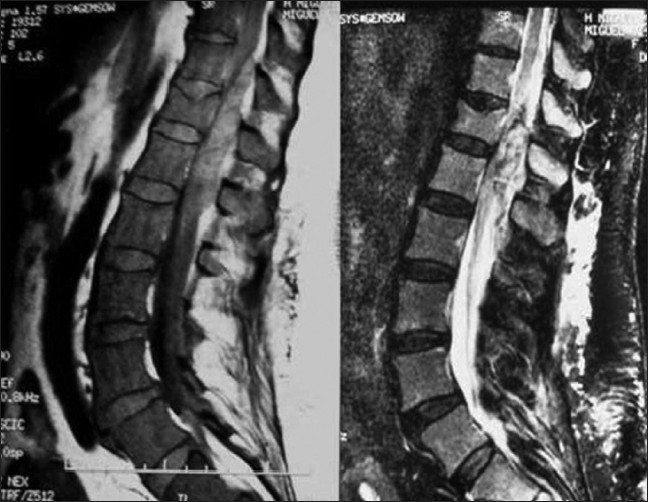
Sagittal MR image of thoracolumbar region showing relatively hyperintense lesion on T1 that becoming further hyperintense on T2 image with compression of the cord extending from D11 to L1 level
